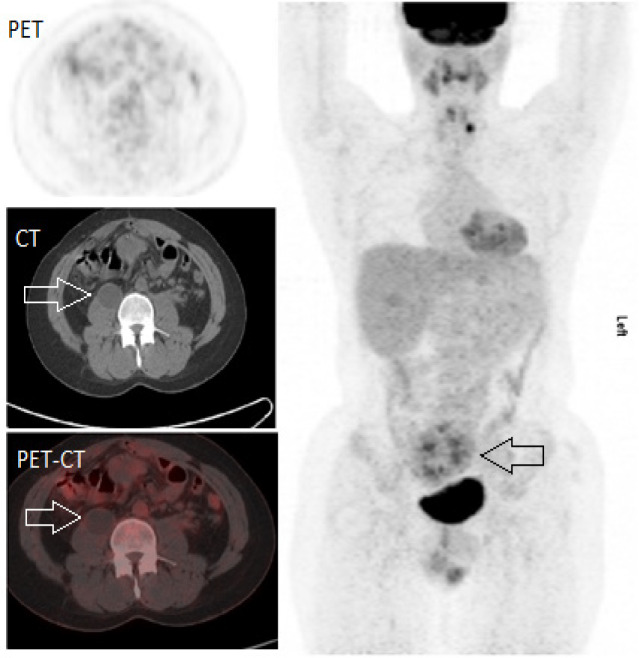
48-Year Old Man Underwent Follow up CT Scan Showing Cystic Lesion at the Right Psoas Muscle (white arrows). 18F-FDG PET CT showed negligible FDG uptake in the cystic lesion consistent with benign lesion
